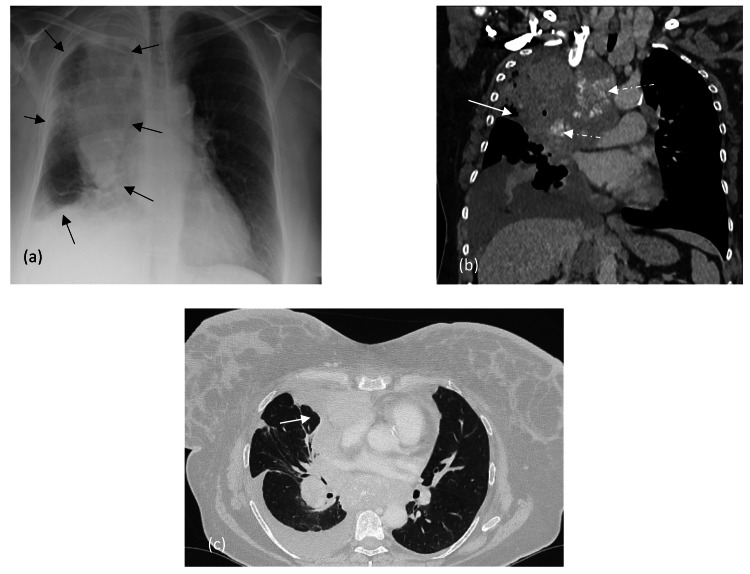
Chest radiographs (a): Circumferential pleural thickening of the right lung (within black arrows) with decreased lung volume. CT chest with contrast (b) and (c): nodular pleural thickening that encases the right lung with extension onto the mediastinal pleura (white solid arrows) and into the fissures. Large calcified pathologically enlarged mediastinal and hilar lymph nodes (white dashed arrows) are seen.
